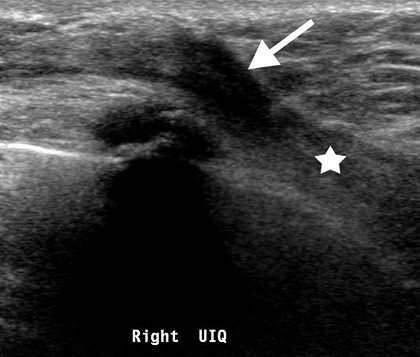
Ultrasound examination showing deep-seated angular hypoechoic lesion measuring 1.5 x 8cm (arrowed) extending to the pectoralis (starred), considered stage 4 due to pectoralis muscle involvement
